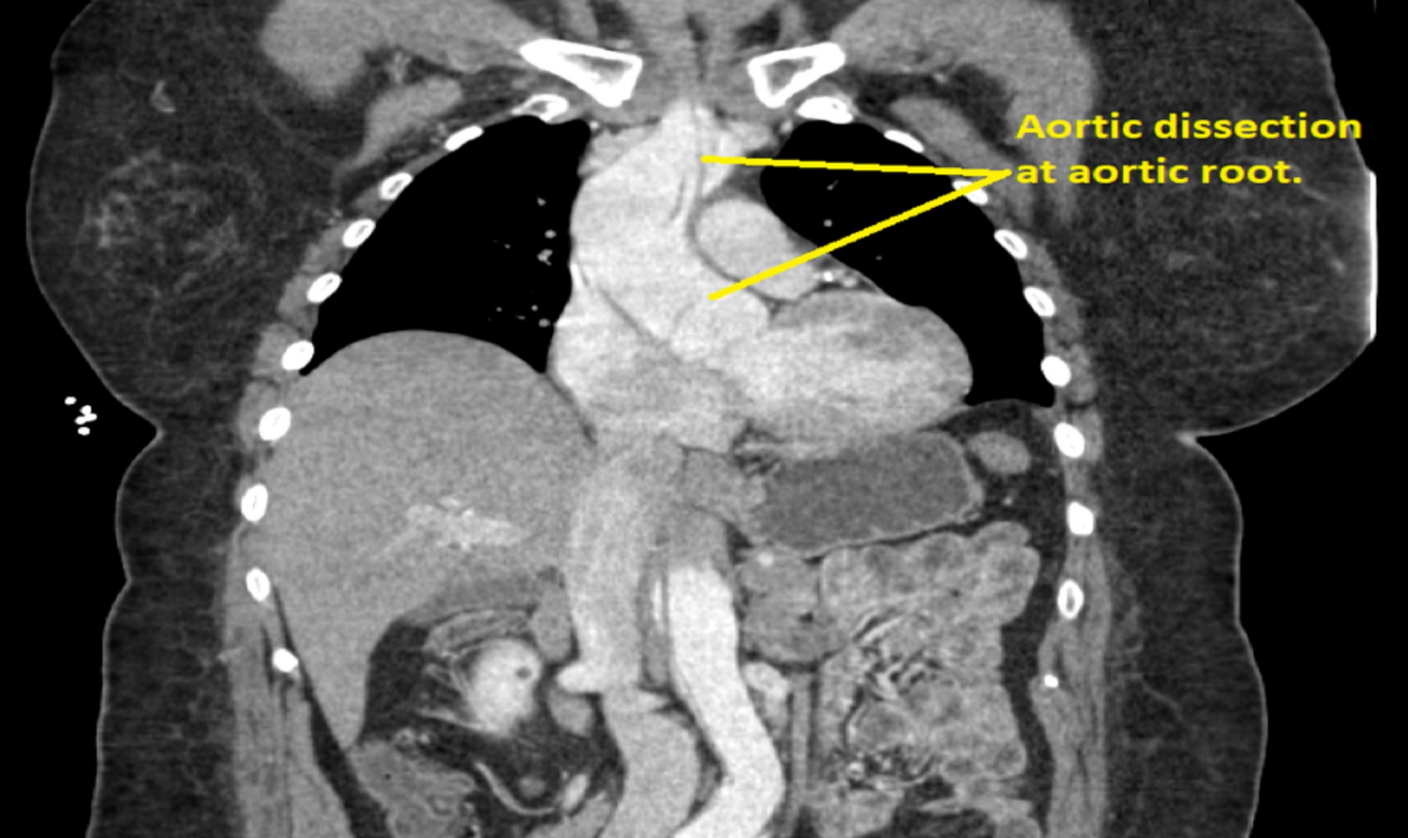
CT scan showing dissection at aortic root.
